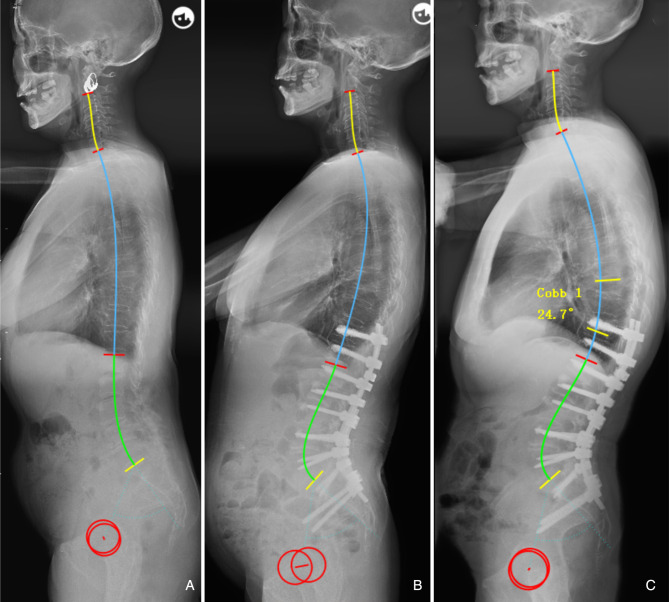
66-year-old female. (A) preoperative sagittal standing full spine X-rays, pelvic incidence (PI) = 57.6°, thoracic kyphosis (TK) = 19.9°, lumbar lordosis (LL) = −36.1°. (B) immediate postoperative X-rays, pelvic incidence = 56.6°, thoracic kyphosis = 27.1°, lumbar lordosis (LL) = −65.1°; ideal LL = 0.7*56.6 + 0.4*27.1 + 1 = −51.5°, ΔLL = 65.1–51.5 = 13.6°. (C) PJK occurred at 6-month follow-up.
